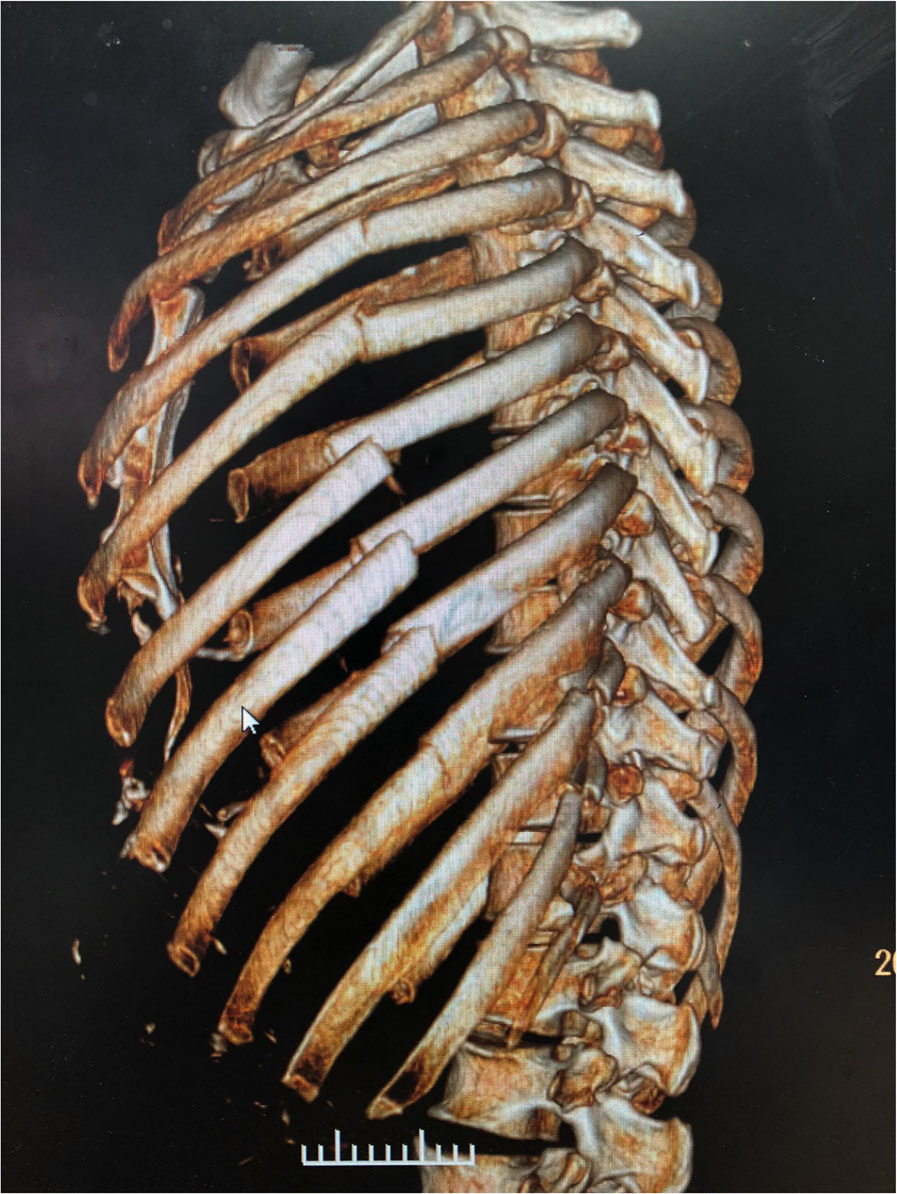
Chest computed tomography and three-dimensional reconstruction of the ribs
